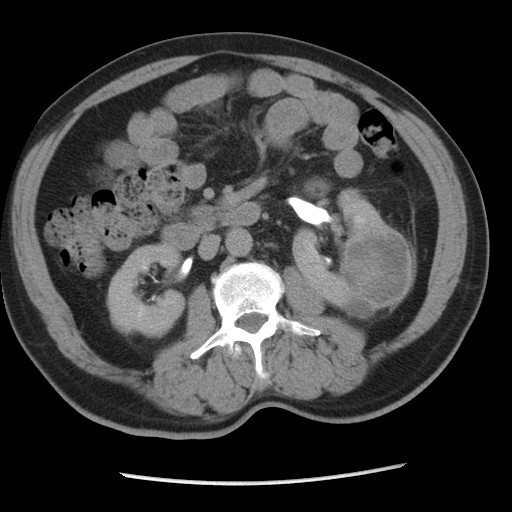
CT scan showing a huge cystic tumor of the left kidney.
